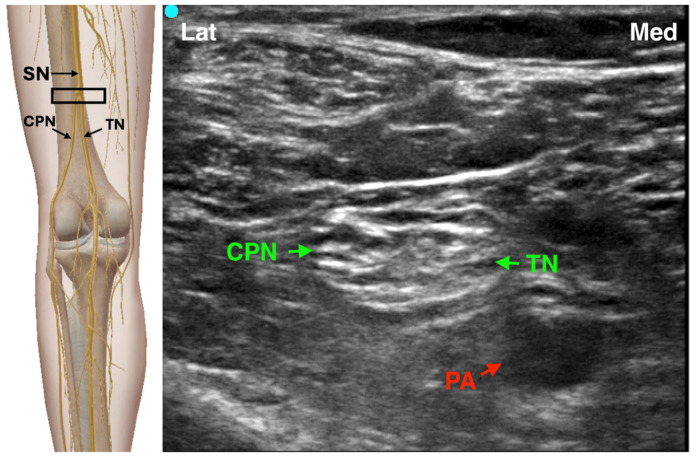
Ultrasound-guided Popliteal Sciatic nerve Block SN: Sciatic Nerve; TN: Tibial Nerve; CPN: Common Peroneal Nerve; PA: Popliteal Artery; The black rectangle indicates the placement of the ultrasound probe on the anatomical area.
